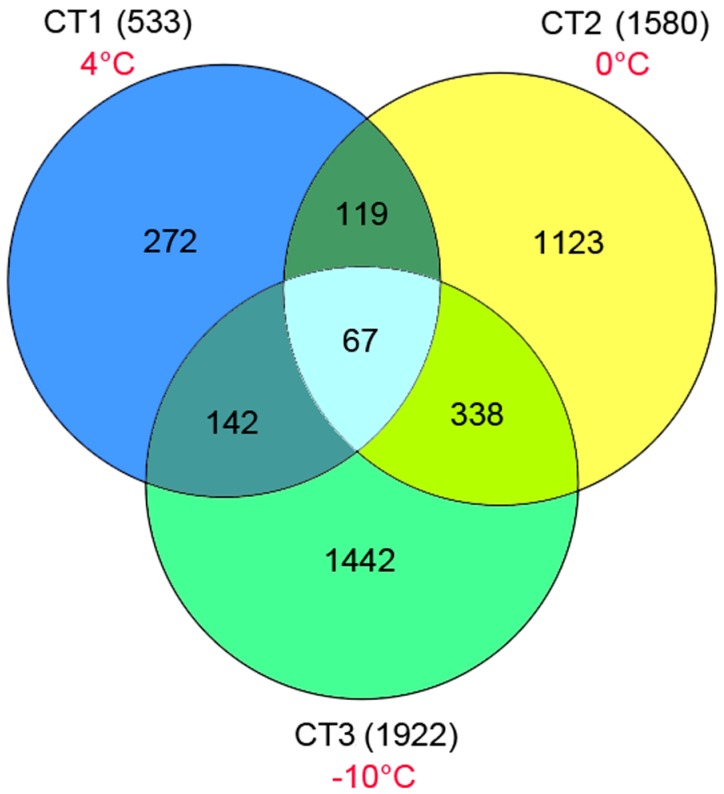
The numbers of up-regulated genes expressed only in the three CT samples (4, 0, and –10 °C).
